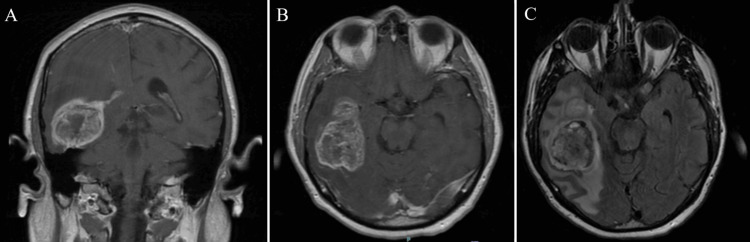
Initial imaging at the time of diagnosis: (A) Coronal and (B) axial T1-weighted MRI with contrast and (C) FLAIR sequence showing a large right temporal mass with significant vasogenic edema
